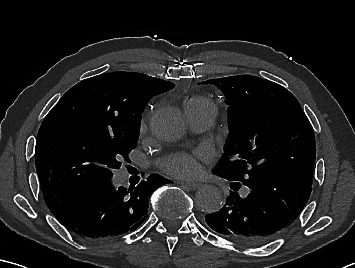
Exemplary transverse section of the staging CT image at initial diagnosis: the number of thoracic lymph nodes is clearly increased.
